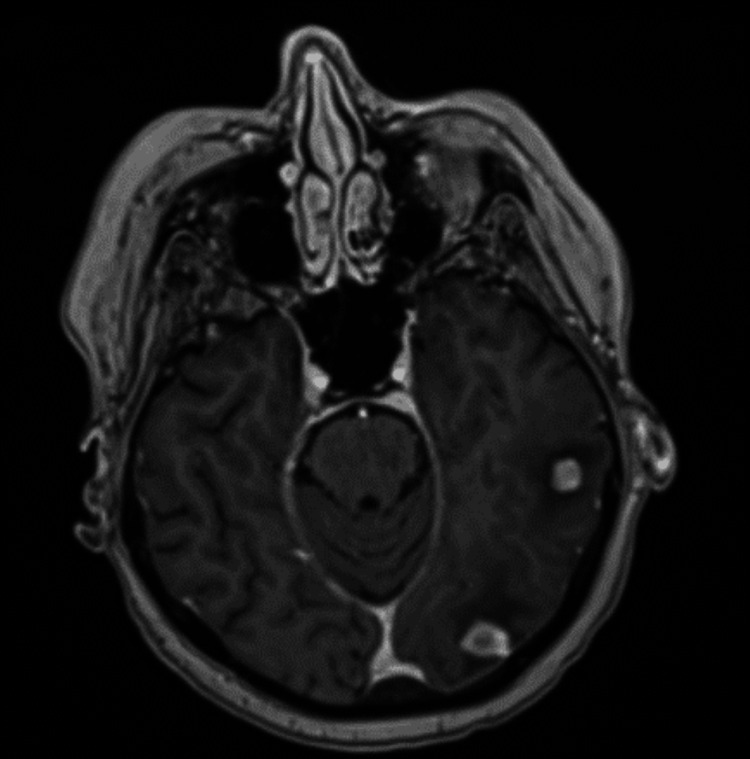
Post-contrast axial T1-weighted magnetization-prepared rapid gradient-echo (MPRAGE) magnetic resonance imaging (MRI) demonstrating a sample of left-sided intracerebral lesions
